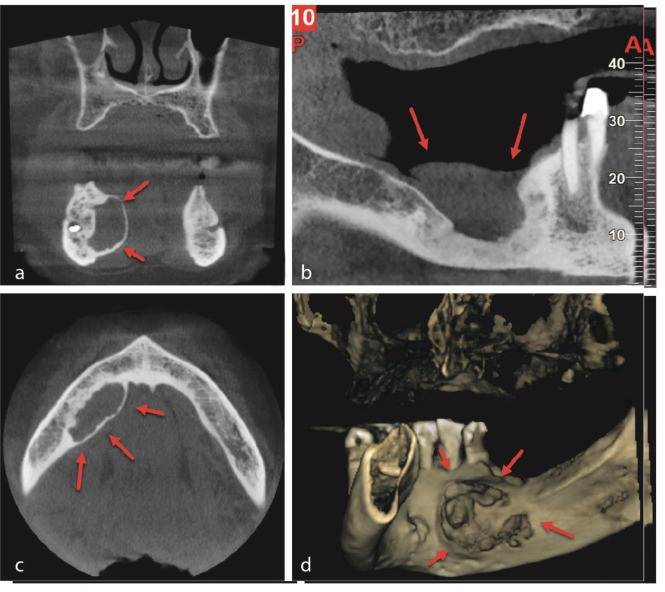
Cone beam computed tomography (CBCT) scans depicting the lesion at various views (Red Arrow); a: Coronal View, b: Sagittal View, c: Axial View, d: Full 3D View
